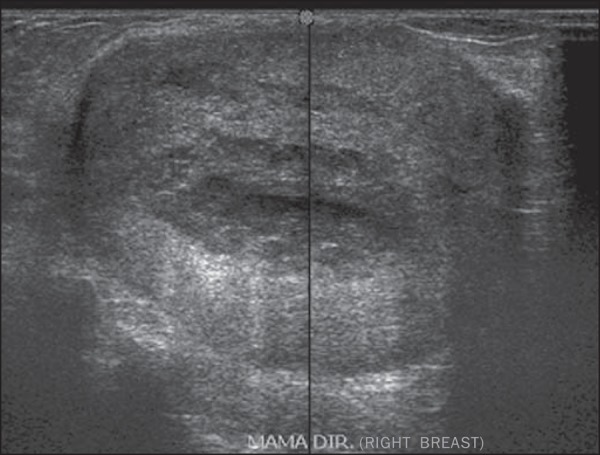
Malignant phyllodes tumor*. Ultrasonography showing
